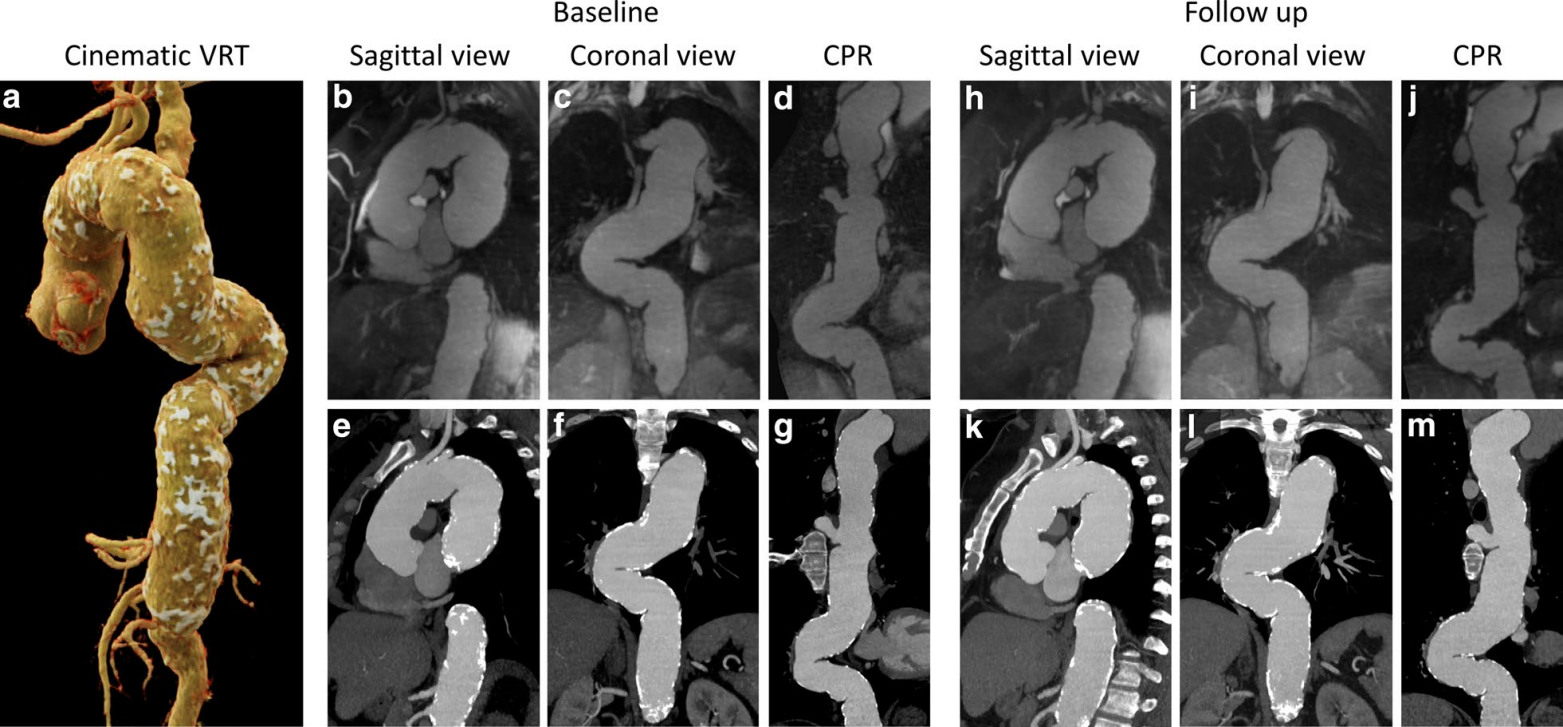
Representative case example demonstrating disease monitoring over a 1-year follow up. Cinematic volume rendering technique (a), generated from the baseline CTA of a 46-year-old woman, shows the overview of an extensively calcified and tortuous aorta. Aneurysmal aortic dilatation is present at the level of the root, ascending and descending aorta, extending to the level of the celiac artery. Corresponding CMRA (top row, b–d and h–j) and CTA (bottom row, e–g and k–m) images in sagittal, coronal and curved planar reformat views are shown at baseline (b–g) and at 1-year follow up (h–m). The maximum diameter increased from 47.4 to 53.0 mm (11.8%) in the ascending aorta, and from 53.2 to 54.3 mm (2.1%) in the descending aorta. *VRT* volume rendering technique, *CPR* curved planar reformat
